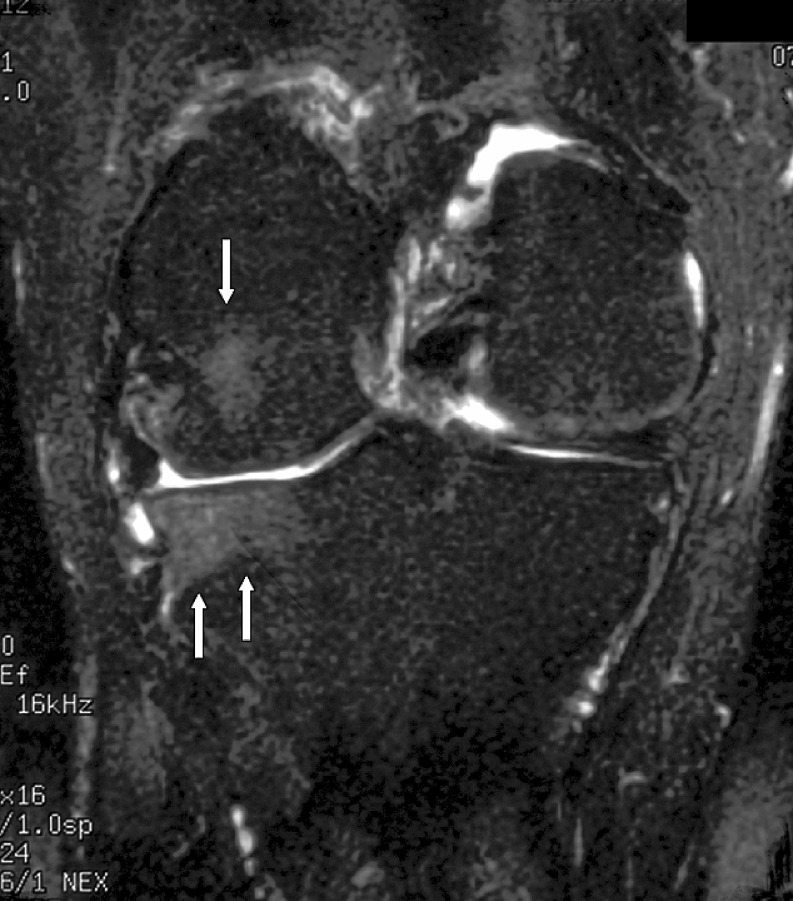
Global edema, no total knee joint replacement, in a 58-year-old male patient. Coronal fast fat suppressed T2-weighted image (TR 3000/TE 76/256 × 256) through the knee shows extensive edema in the lateral tibial condyle and edema in some of the lateral femoral condyle (*arrows*). Note also the almost complete absence of hyaline cartilage
